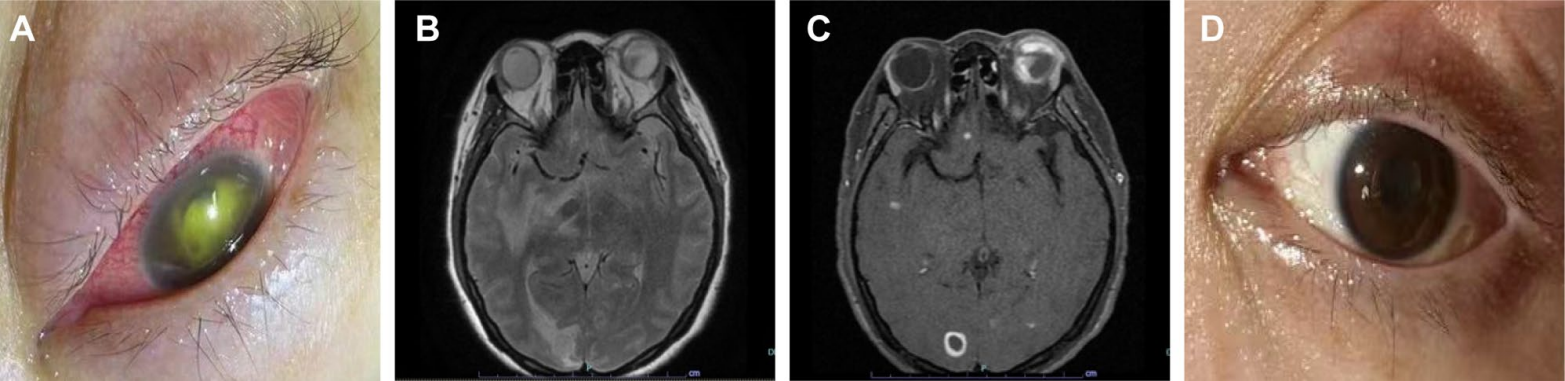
Ocular appearance and orbital MRI. (A) One month after the accident, left eye conjunctival congestion, anterior chamber visible numerous yellow-green exudates sealed the pupil. (B, C) On March 22, 2023 (3 weeks of antifungal treatment), an orbital MRI showed an abnormal signal of the left eyeball with enhancement. (D) On April 6, 2023(5 weeks of antifungal treatment), the pupil of the left eye was clear, but the eyeball atrophy and vision loss remained
